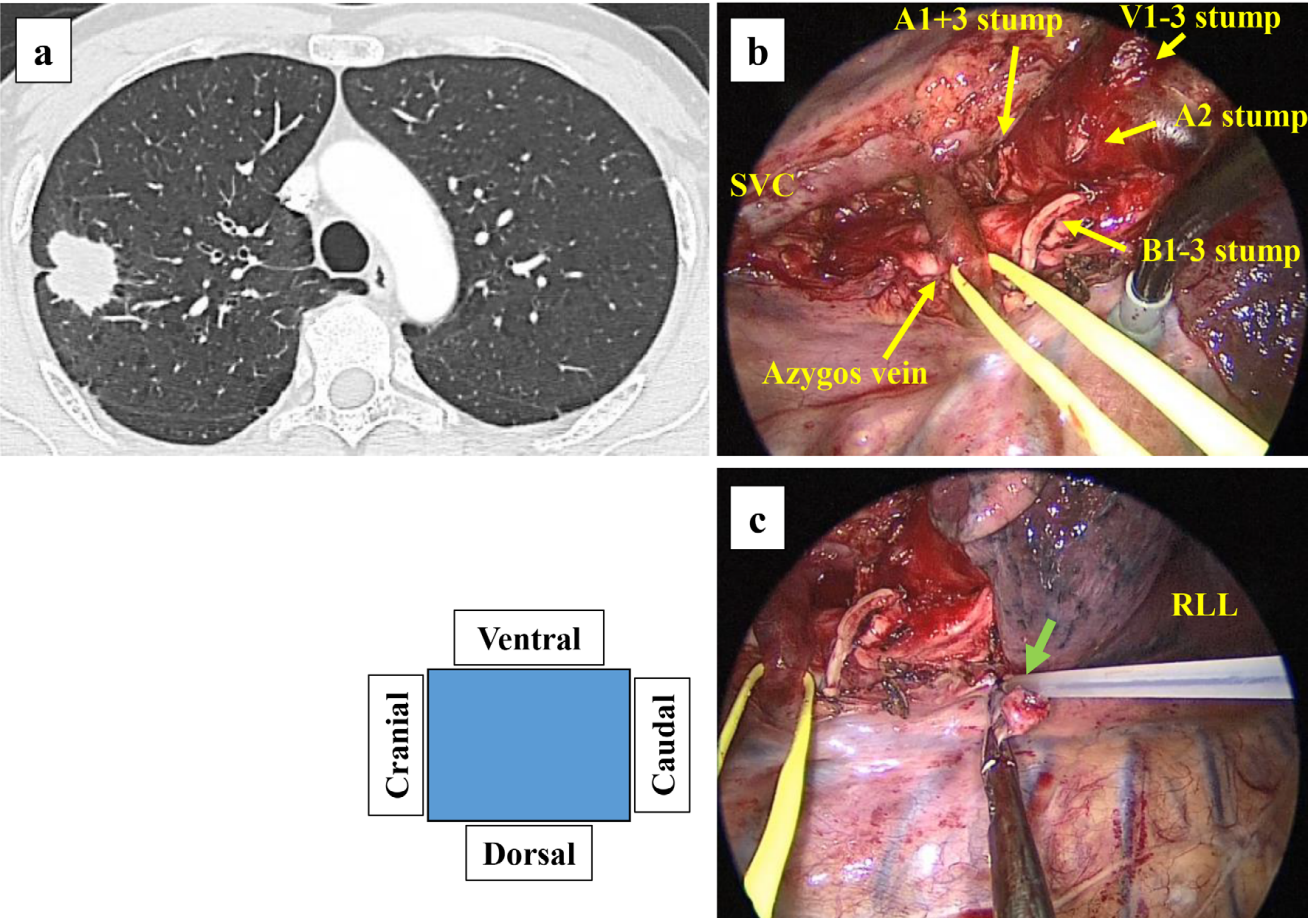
Preoperative computed tomography image and surgical findings of right upper lobectomy. (a) Computed tomography (CT) scan revealed lung cancer in the right upper lobe and emphysema mainly in the bilateral upper lobes. (b) Surgical findings after right upper lobectomy. (c) A pulmonary cyst at the top of the right sixth segment was ligated (green arrow). RLL, right lower lobe; SVC, superior vena cava.
